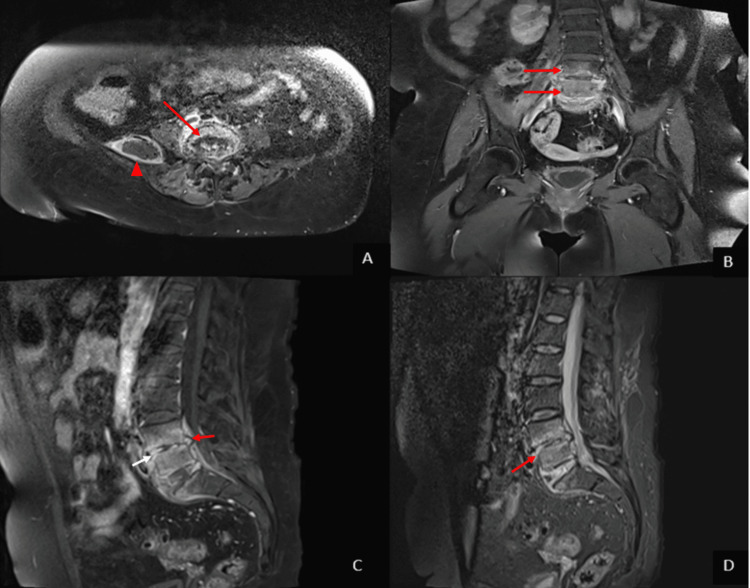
Multimodal MRI findings of L4-L5 infectious spondylodiscitis. (A) Axial T2-weighted fat-saturated image: High-signal intensity in the disc space (red arrow) and a well-defined fluid collection in the right paravertebral space (arrowhead), confirming an organized abscess rather than a diffuse phlegmon. (B) Coronal T2-weighted STIR image: Extensive bone marrow edema in the L4 and L5 vertebral bodies (red arrows), with inflammatory infiltration extending toward the right psoas muscle. (C) Post-contrast sagittal T1-weighted fat-saturated image: Anterior epidural involvement (epiduritis) is visible (red arrow) in continuity with the infected L4-L5 disc space (white arrow). Despite this extension, there was no significant spinal canal stenosis or neural compression, correlating with the lack of neurological deficits. (D) Sagittal T2-weighted fat-saturated image: Diffuse hyperintensity within the L4-L5 disc and adjacent vertebrae (red arrow), associated with grade I anterolisthesis of L4 on L5. STIR: Short tau inversion recovery.
